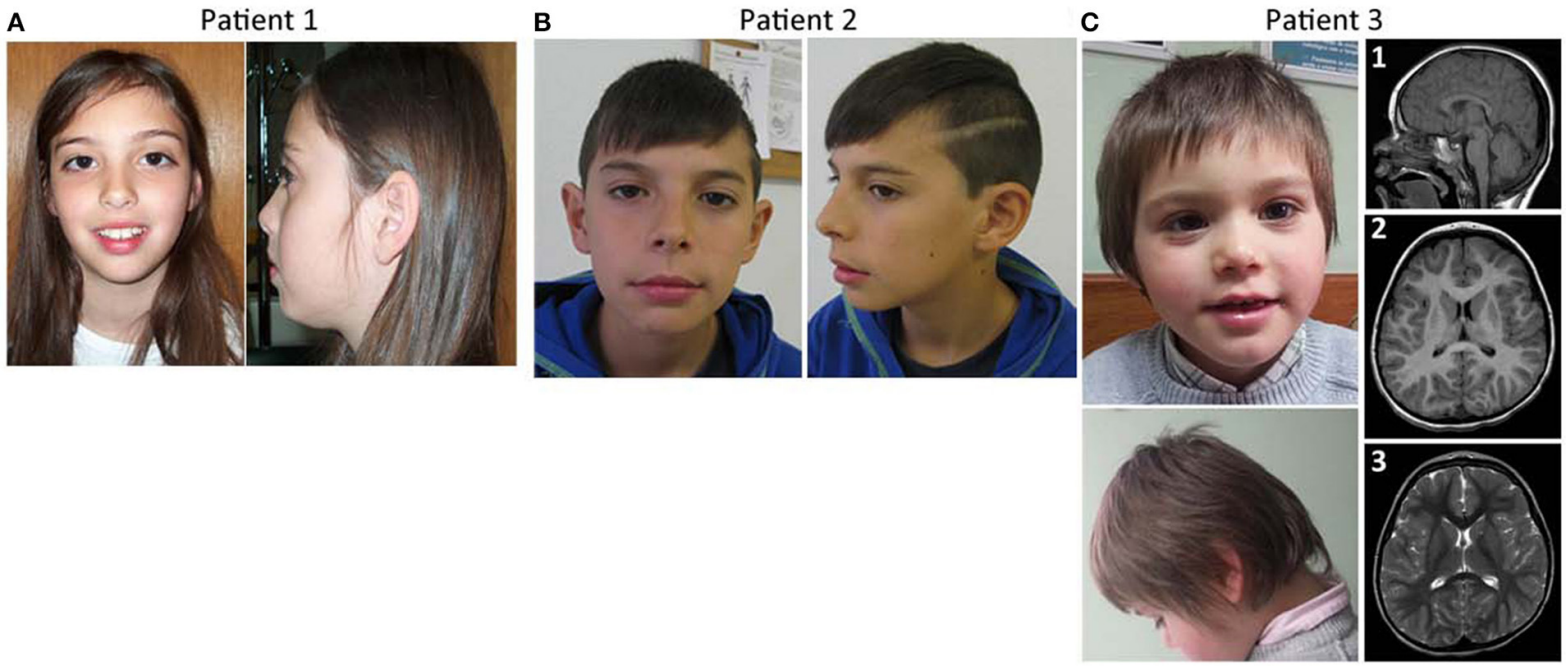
Facial features and brain imaging of the patients. (A) Patient 1 facial features, (B) patient 2 facial features, (C) Patient 3 facial features, and MRI brain imaging.
